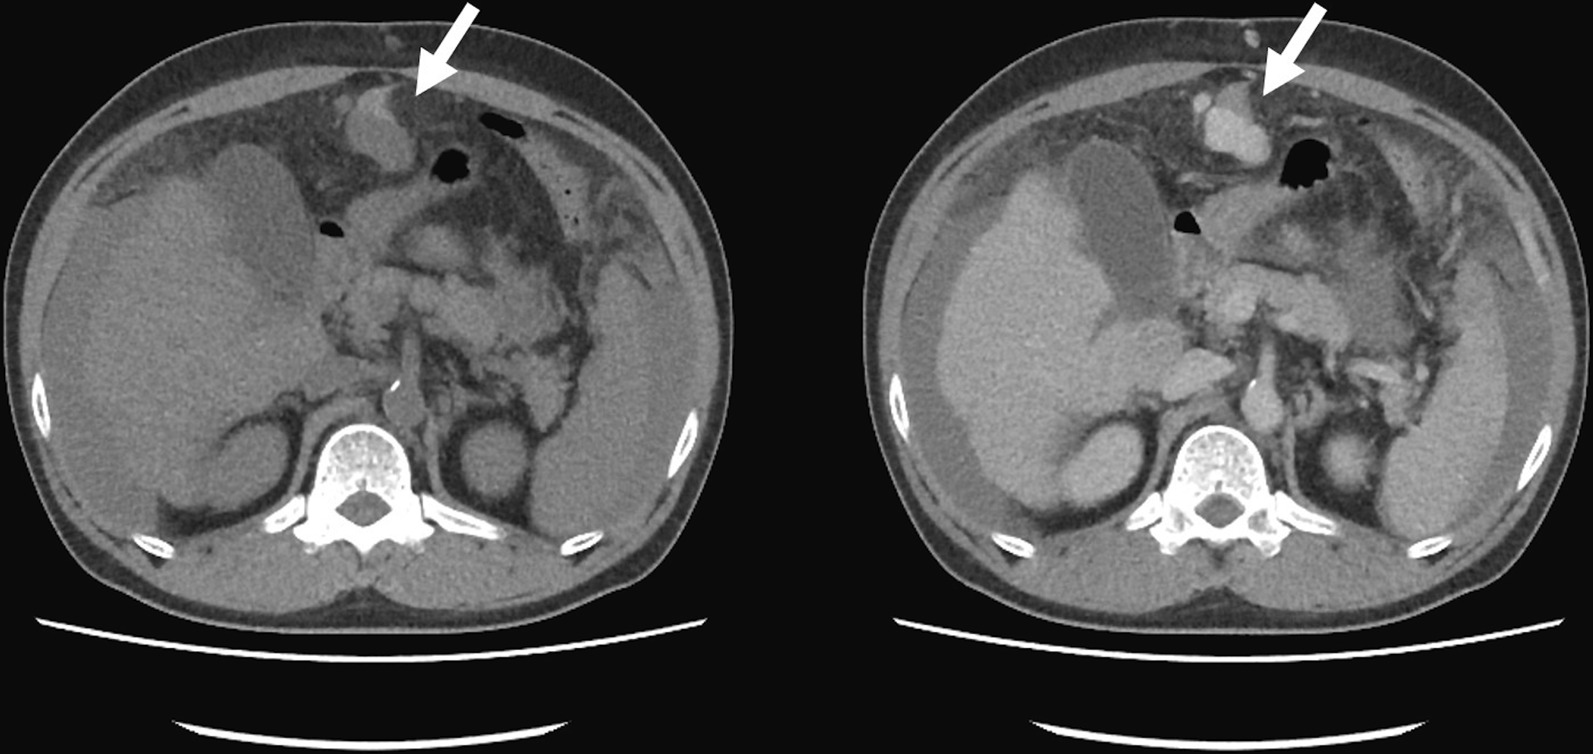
Abdominal CT demonstrated a dilated tubular structure originated from falciform ligament with contrast enhancement. In non-contrast phase, there was an adjacent high-density content beside umbilical area
